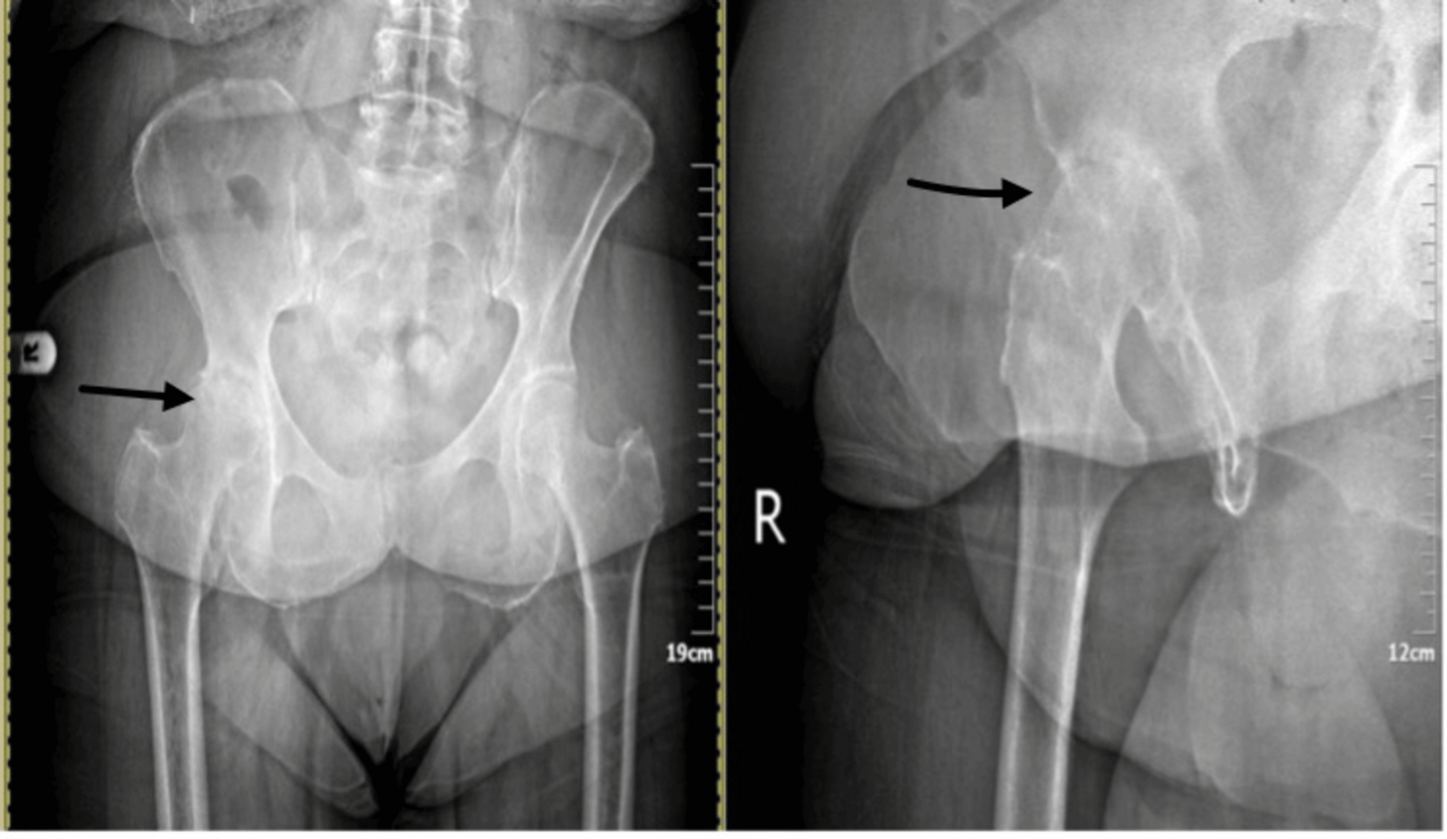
Preoperative plain radiographs
